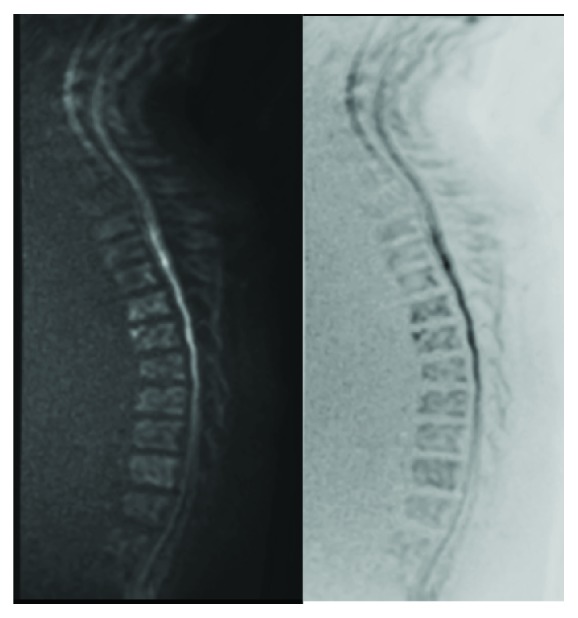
Diffusion-weighted MRI slightly showing abnormality on day 5 (a). Signal changes could be seen a little more clearly on a black and white inverted image (b).
